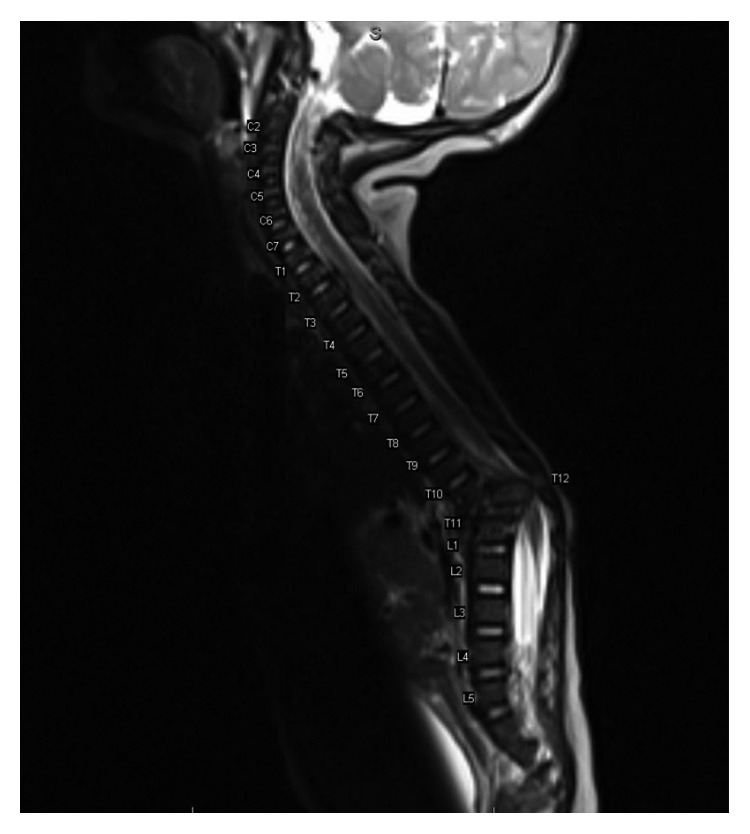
MRI of the spine with separation/avulsion of the T12 vertebral body from the T12 posterior elements. The T12 vertebral body appears to be rotated counterclockwise on axial image and displaced posteriorly into the bony spinal canal resulting in marked compression of the spinal cord along with signal abnormality in the spinal cord just inferior and superior to the compressed T12 segment.
